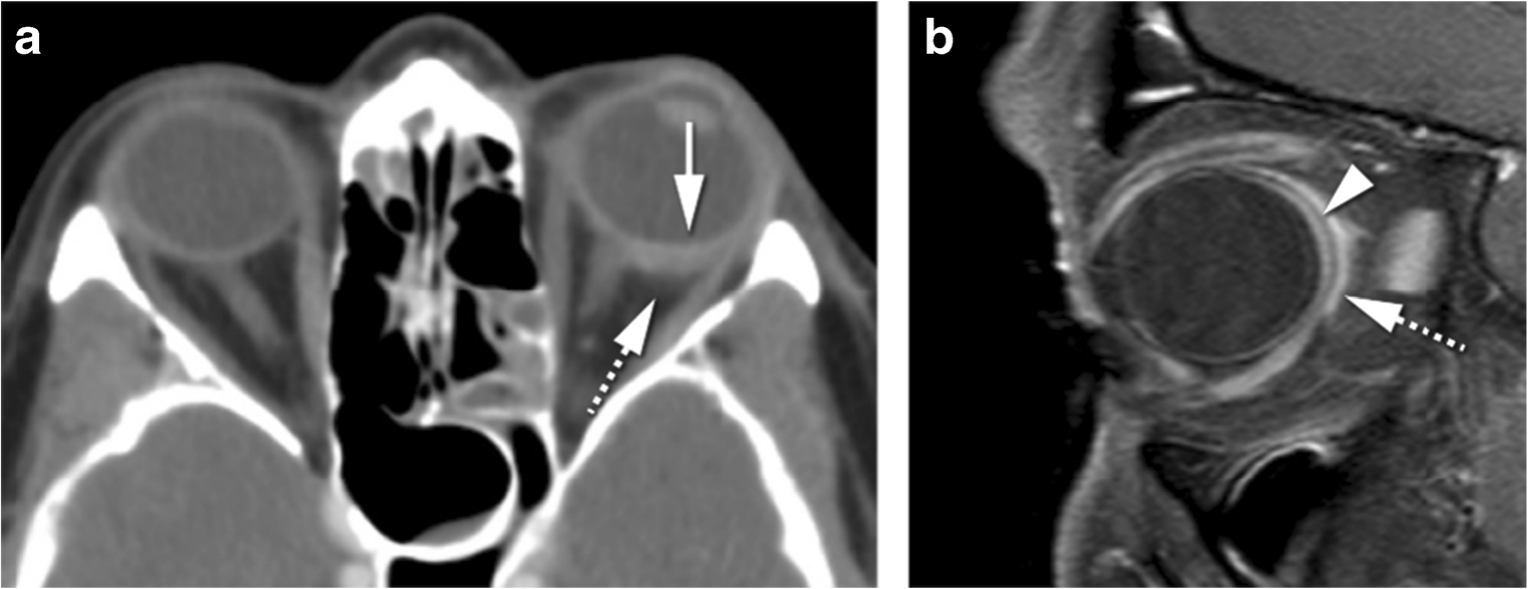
a 69-year-old female. Inflammatory scleritis on the left. Axial contrast enhanced CT (CECT): focal eccentric outward thickening and enhancement of the globe wall (arrow) and minimal blurring of the adjacent fat (dashed arrow), both consistent with scleritis. b 38-year-old male. Idiopathic inflammatory scleritis on the right. Enhanced sagittal T1-WI with fat signal suppression: scleral thickening and enhancement (dashed arrow) and slight suprachoroidal effusion (arrowhead), consistent with scleritis. Contrary to CT, MRI clearly depicts which ocular layer is enhancing
